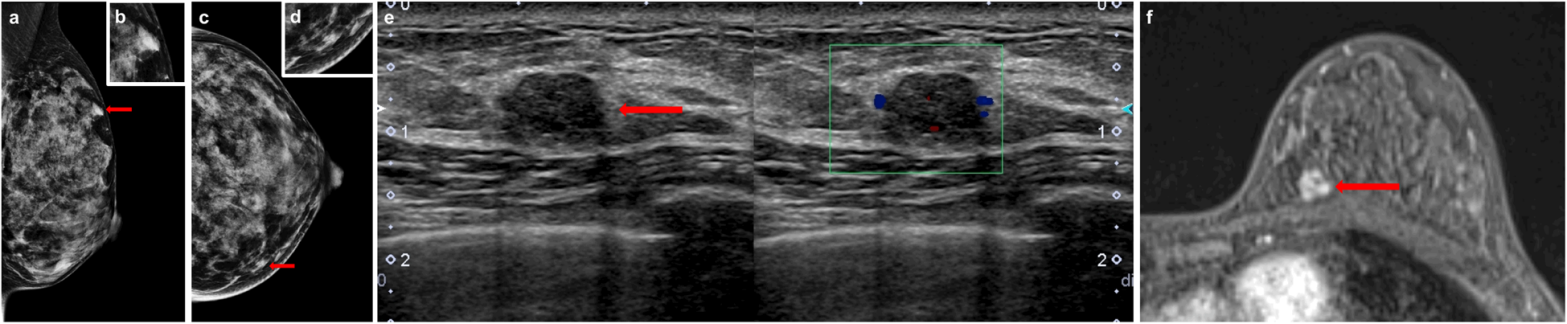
Imaging findings. Mammogram showing a round, high-density mass in the left MLO-M area (a) and CC-I area (c). b, d Enlargements of the areas are indicated by red arrows. US shows a regular, circumscribed, hypoechoic, homogenous lesion with abundant blood flow in the A area of the left breast (e). Dynamic MRI demonstrates an ill-demarcated mass that measures 8 mm × 7 mm in the A area of the left breast (f) without daughter lesions or enlarged lymph nodes
